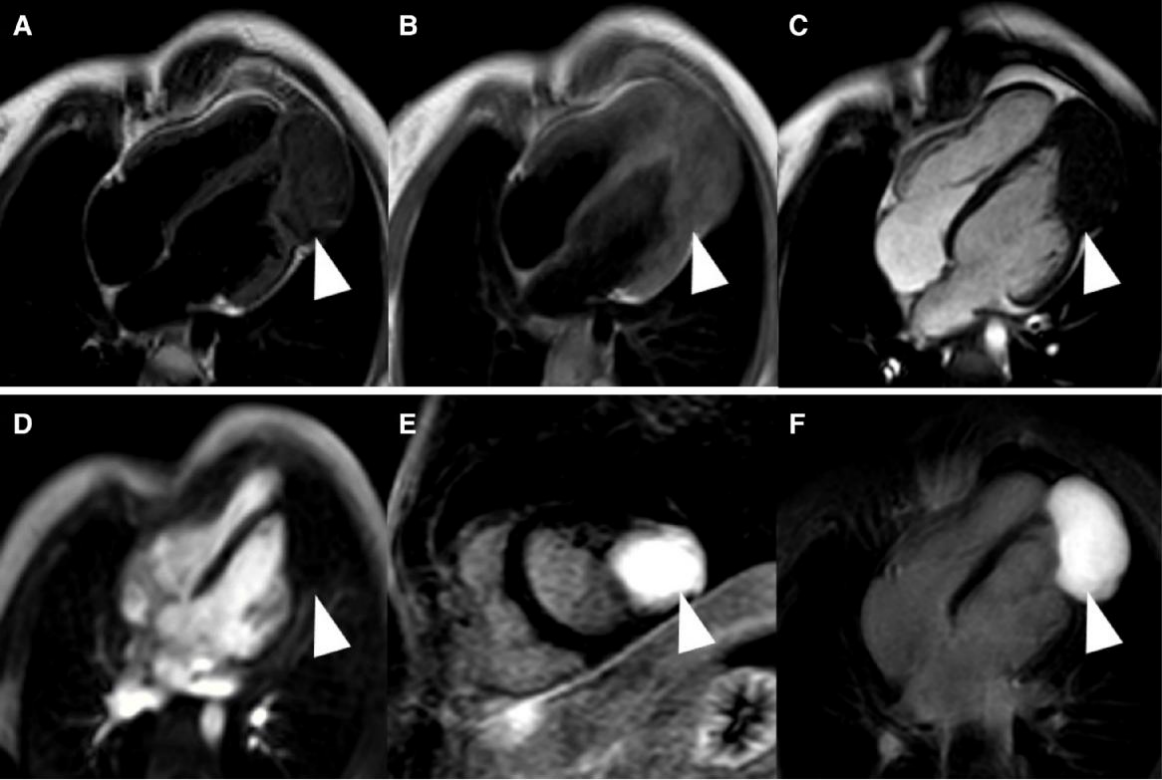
Cardiovascular magnetic resonance showing the fibroma of left ventricular apex with an iso- to hypointense signal in T1- (*A*, arrowhead) and iso- to hypointense signal in T2-weighted (*B*, arrowhead) sequences in four-chamber view. Cine sequences in four-chamber view revealed an iso- to hypointense signal (*C*, arrowhead). There was no hypervascularization of the mass in the first-pass-perfusion study in four-chamber view (*D*, arrowhead). Late gadolinium enhancement revealed a homogeneous enhancement in short axis (*E*, arrowhead) and four-chamber view (*F*, arrowhead) without calcifications.
